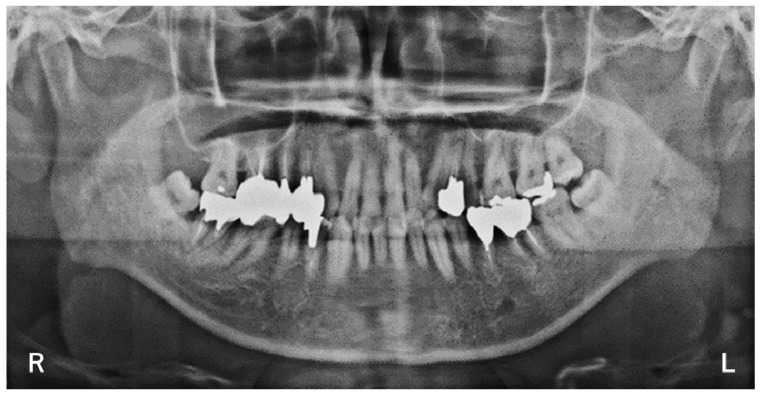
A panoramic radiographic view of the TMJ showed a normal shape in both condyles. R: right. L: left.
